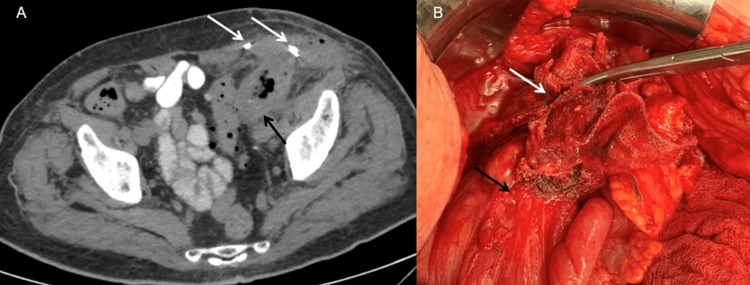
(A) Abdominal CT scan reveals an abdominal abscess communicating with the sigmoid colon (black arrow) and mesh fixating materials identified in the left inguinal area. (B) Intraoperative photograph indicating sigmoid colon erosion (black arrow) by left inguinal mesh (white arrow).
